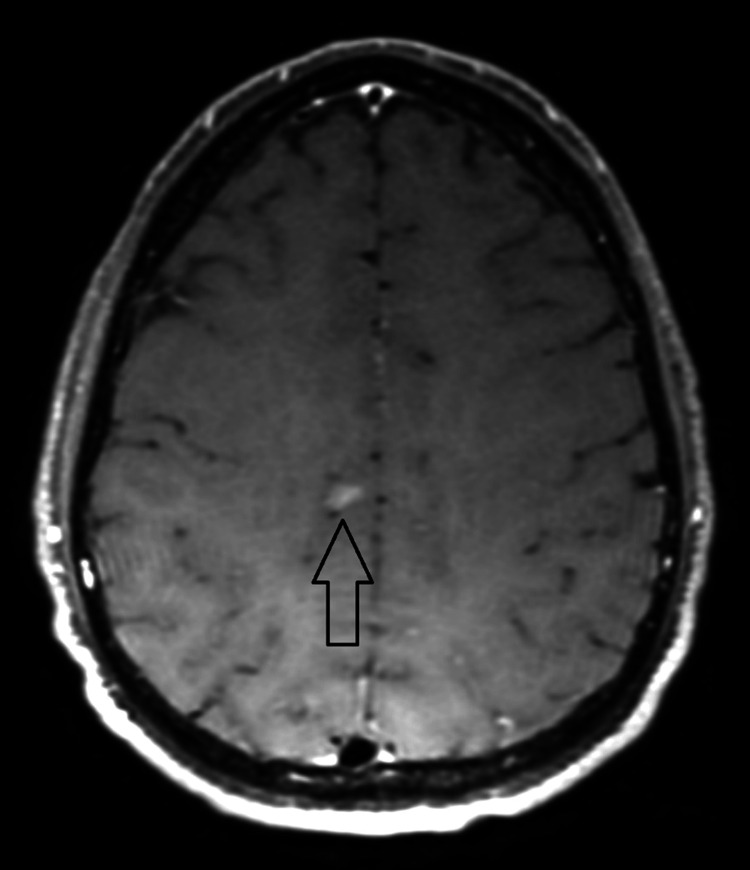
One of the two (nonspecific) enhancing brain lesions demonstrated by contrast-enhanced axial T1 weighted MRI
